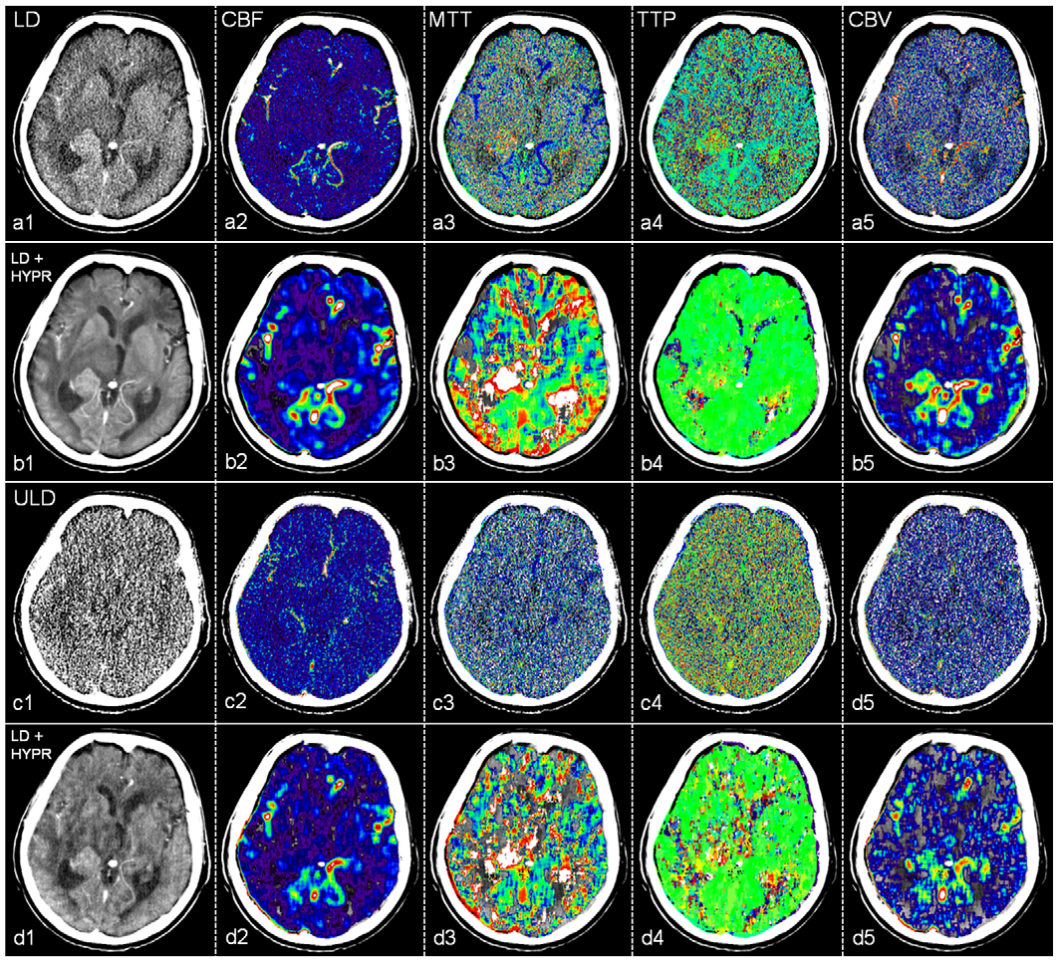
Low dose (LD, a), HYPR-LR-post-processed low dose (LD+HYPR, b), ultra low dose (ULD, c) and HYPR-LR-post-processed ultra low dose (ULD+HYPR, d) brain perfusion CT of a 75-year old male patient with a lung cancer metastasis adjacent to the right thalamus and chronic left frontal infarction. Last image of the 60 s time series (1) and the normalized cerebral blood flow (CBF, 2), mean transit time (MTT, 3), time to peak (TTP, 4), cerebral blood volume (CBV, 5) maps. The utilized software does not use reduced matrix reconstructions or spatial smoothing, the images are left noisy. The pathology is recognizable in a, b and d with excellent subjective image quality and low noise in b. No diagnosis possible in c.
